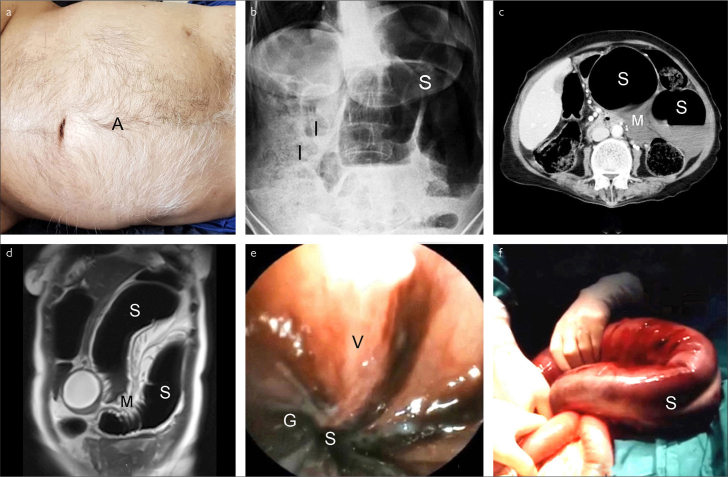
(a) Clinical appearance (A, asymmetrically distended abdomen), (b) Plain erect abdominal X-ray image (S, twisted sigmoid colon; I, intestinal air-fluid levels), (c) Axial abdominal CT image (S, dilated sigmoid colon; M, mesenteric whirl sign), (d) Coronal abdominal MRI image (S, dilated sigmoid colon; M, mesenteric whirl sign), (e) Endoscopic appearance (S, spiral sphincter-like twisted sigmoid lumen; V, viable mucosa; G, gangrenous mucosa), (f) Operative appearance (S, twisted, distended and gangrenous sigmoid colon).
